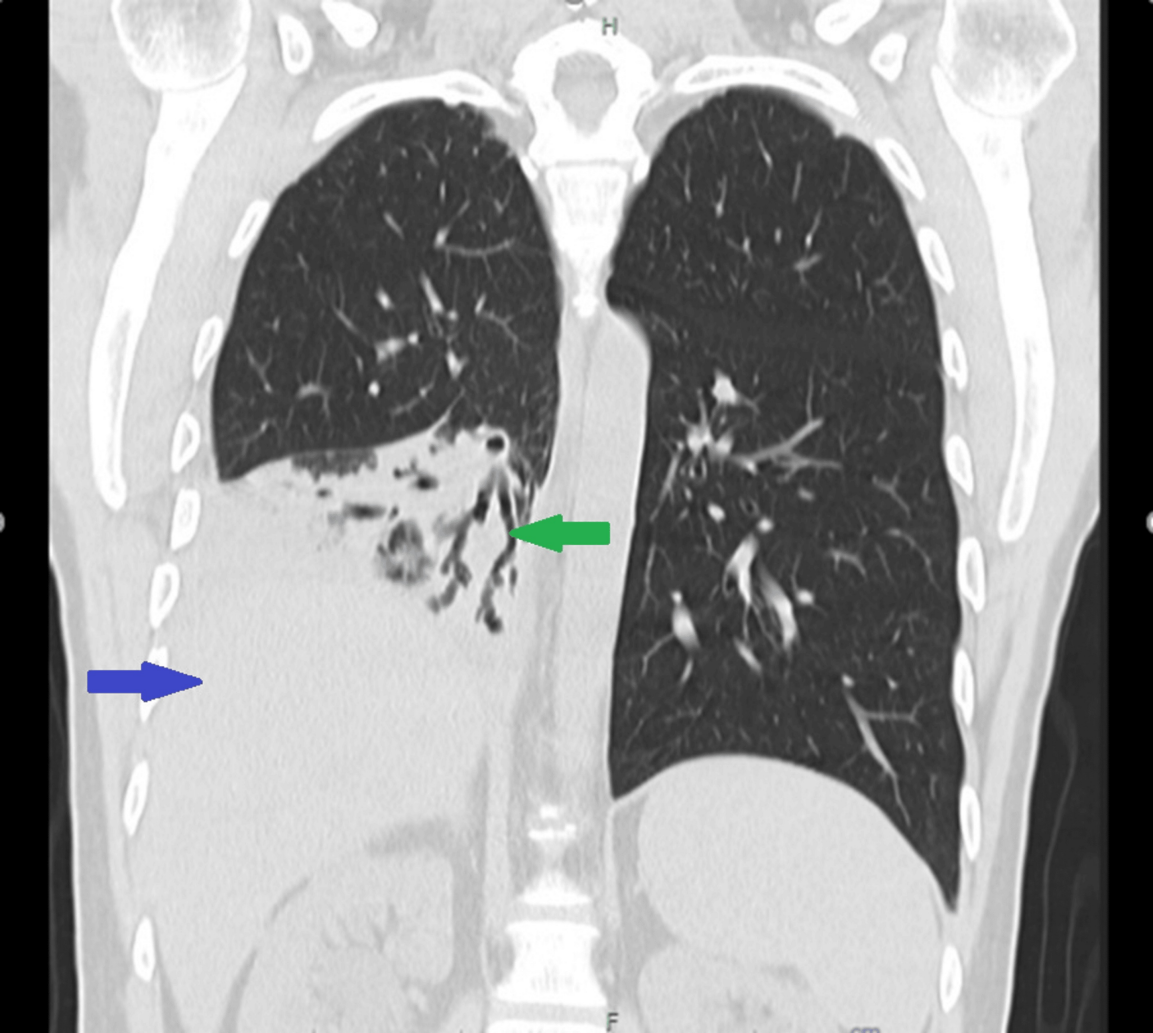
Lung CT scan showing right lobe condensation (green arrow) and pleural effusion (blue arrow)
